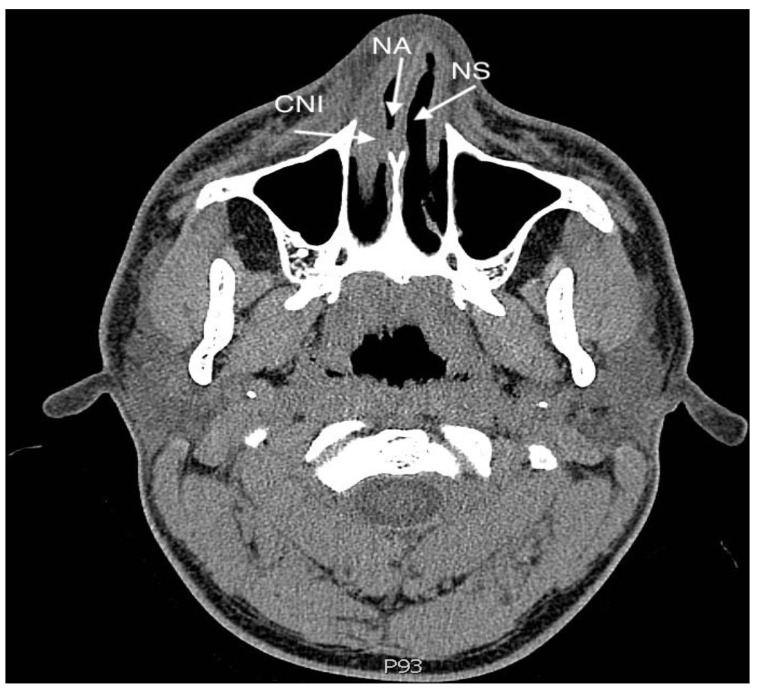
Facial computed tomography scanning of. An adhesion is present between the inferior nasal turbinate and the nasal septum, right side. NS—Nasal septum, CNI—concha nasalis inferior (inferior turbinate), NA—nasal adhesion.
